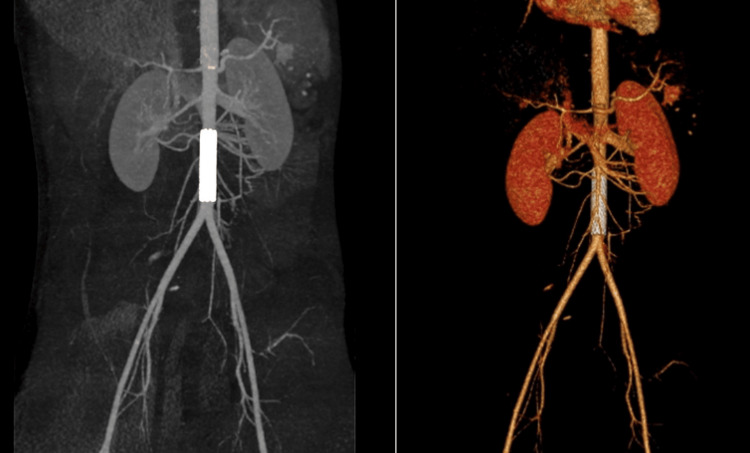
Postoperative computed tomography-angiography verifying the right placement of the endovascular aortic stent.
